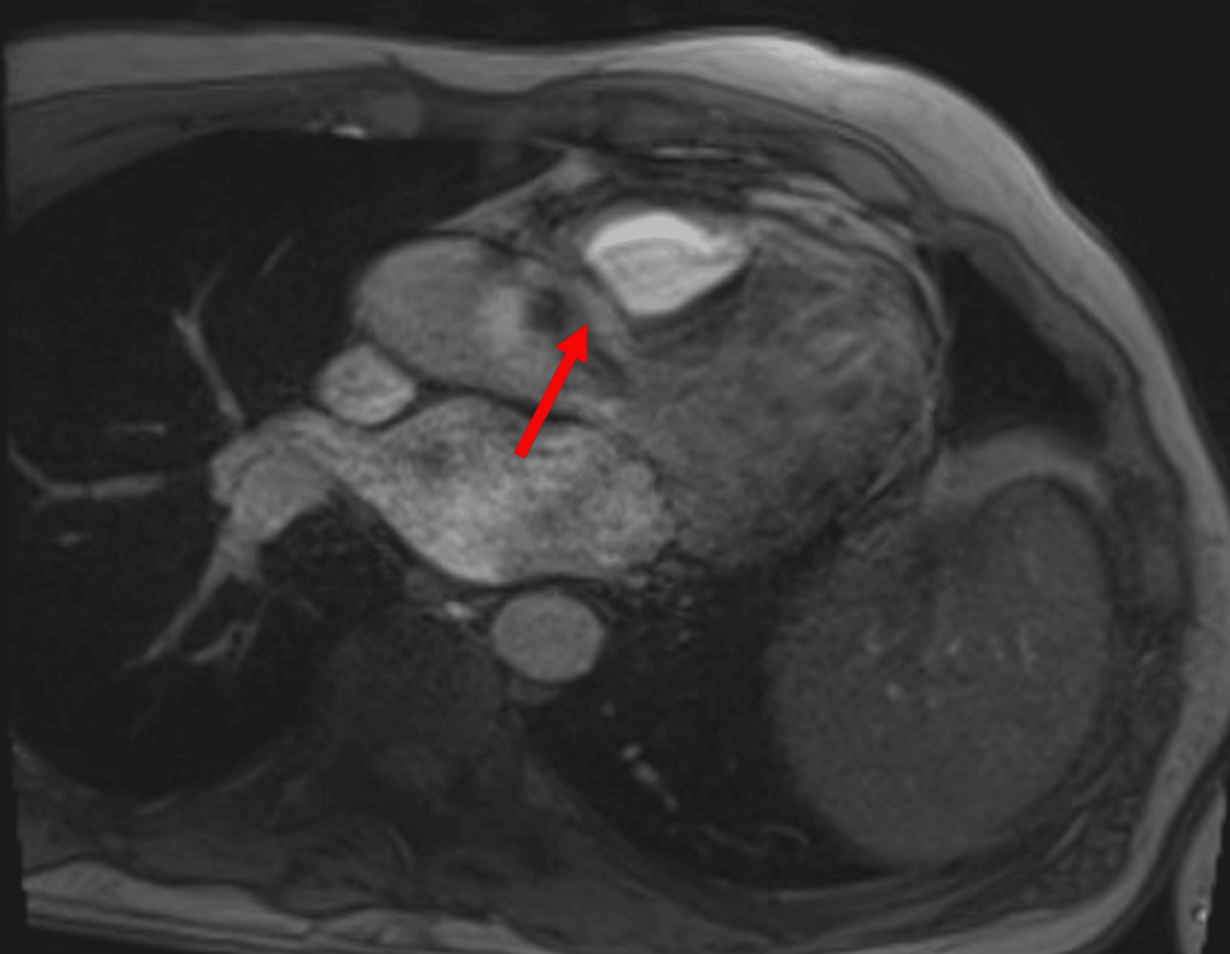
Repeat cardiac MRI showing a mild interval decrease in the right ventricular mass after the first cycle of R-EPOCH R-EPOCH: rituximab, etoposide, prednisone, vincristine, cyclophosphamide, and doxorubicin.
